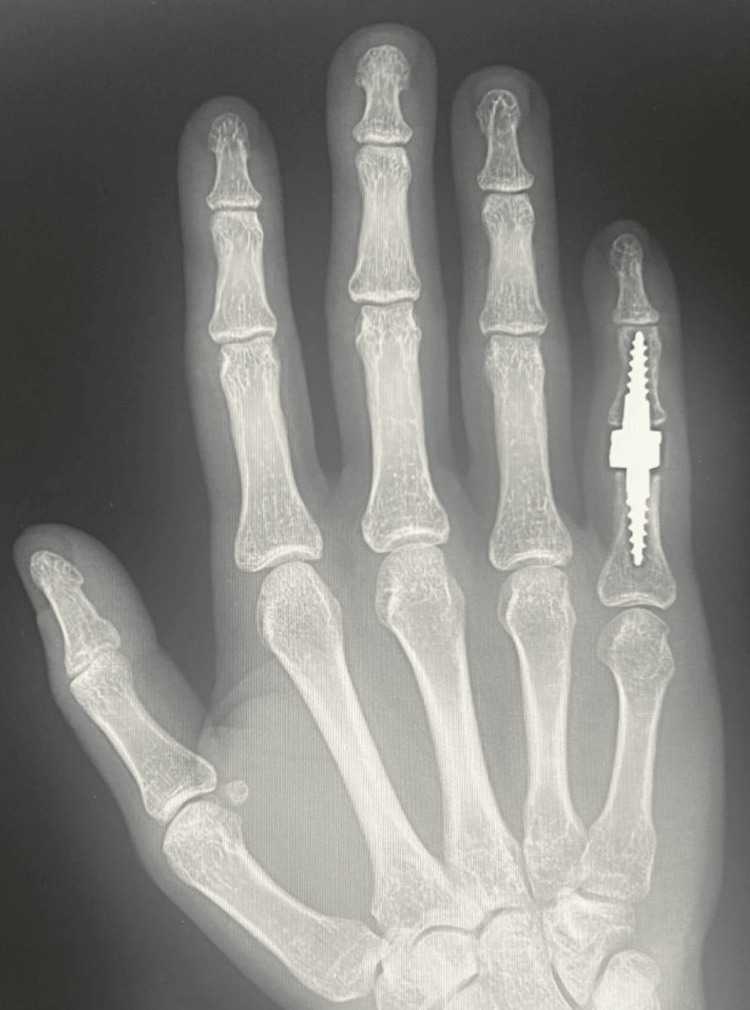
Right hand X-ray in AP projection at week 21 of follow-up
